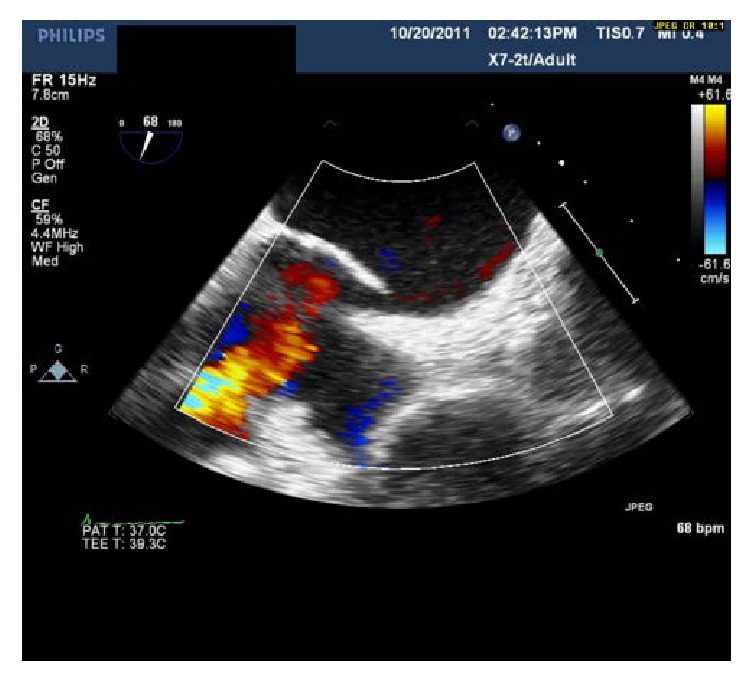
Transesophageal echocardiogram with Doppler demonstrating right-to-left interatrial shunting through the patent foramen ovale.
